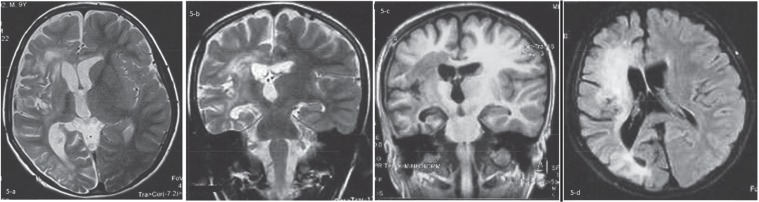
The third brain MRI has done 6 months after biopsy, demonstrated volume loss in the right hemisphere with secondary ventricular prominence. Mass like lesion in the second brain MRI in the right frontal lobe could not be seen any longer (a,b) and abnormal periventricular white matter signal would suggest diffuse gliotic changes or less likely sequel of previous demyelinating lesion (c,d
